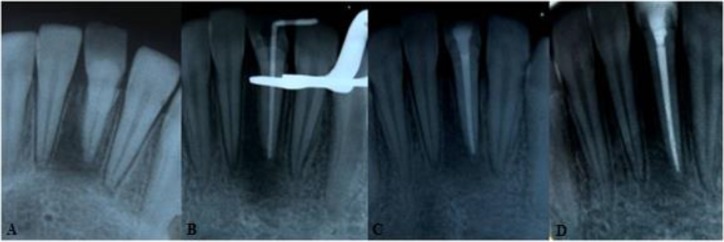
Images showing (A) preoperative X-ray, (B) X-ray during treatment, (C) postoperative X-ray and (D) one-year follow-up
